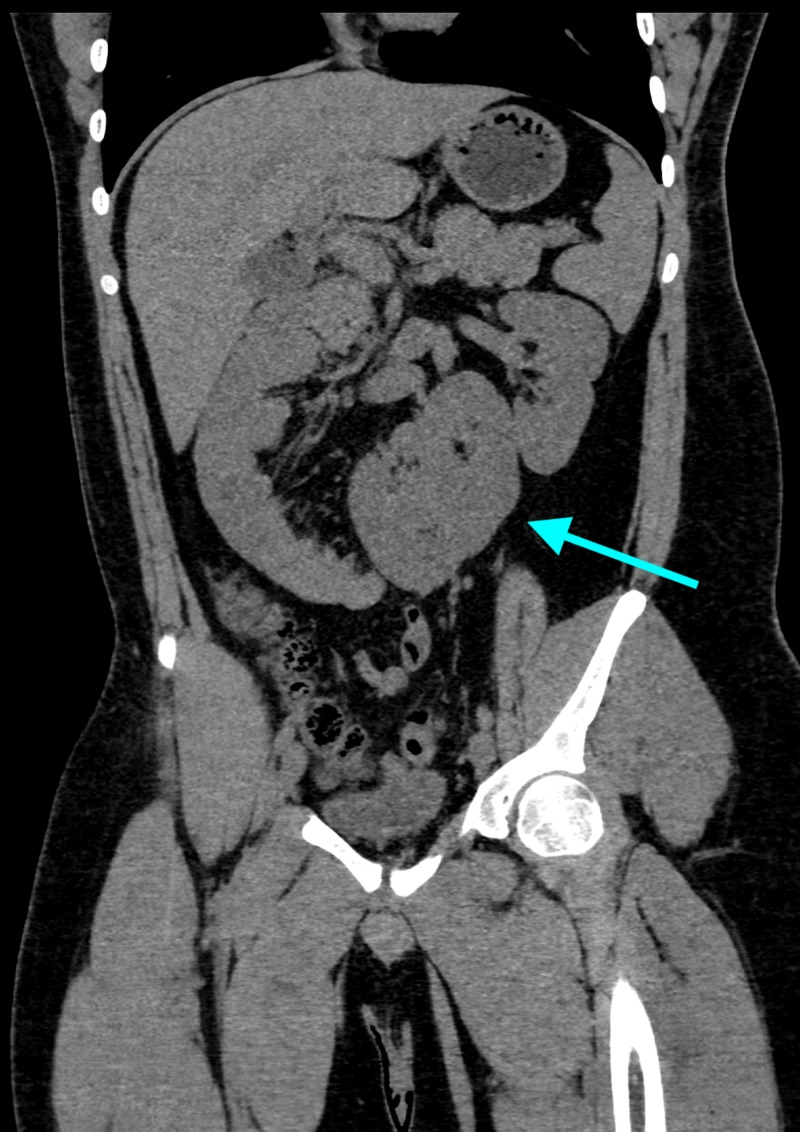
Oblique coronal image from noncontrast CT obtained on the day of presentation demonstrating a normal orthotopic left kidney and a fused ectopic moiety (arrow) abutting the inferomedial aspect of the left kidney consisting of the right kidney and a fused supernumerary kidney CT, computed tomography
